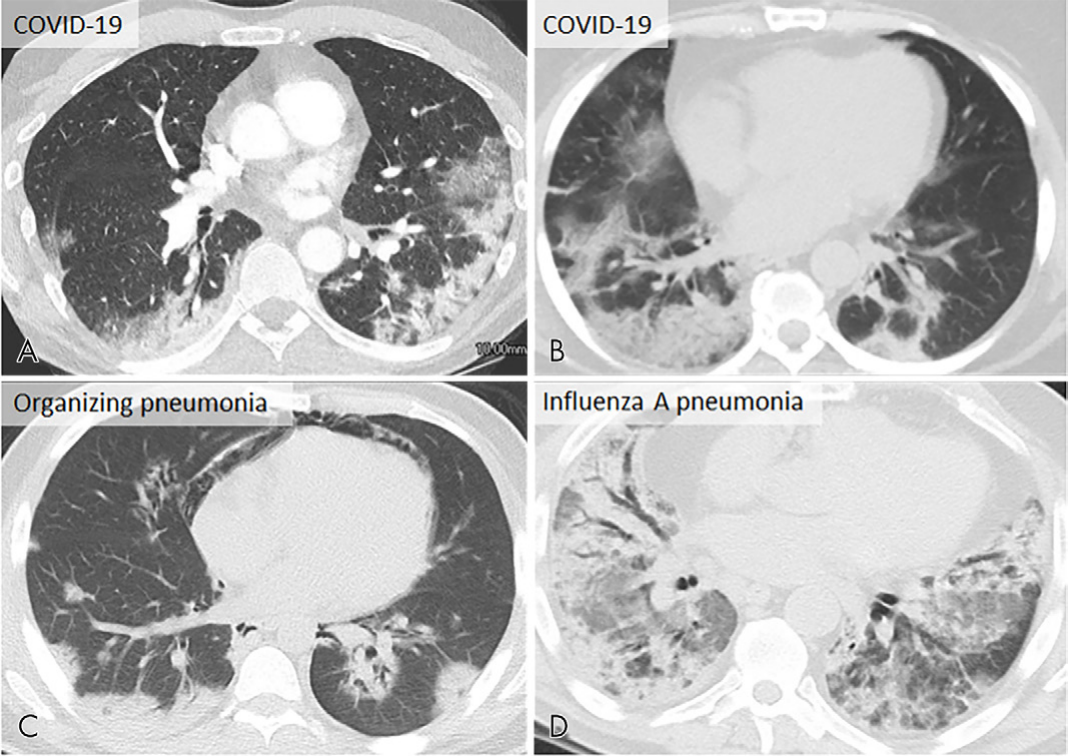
Typical CT imaging features for COVID-19 and other diseases with similar findings. Posterior, peripheral, and rounded GGO and consolidation in axial images of four patients; COVID-19 (*A, B*), organizing pneumonia secondary to dermatomyositis (*C*), and influenza A pneumonia (*D*). Organizing pneumonia and influenza pneumonia can be indistinguishable from COVID-19 by CT. Routine screening CT for diagnosis or exclusion of COVID-19 is currently not recommended by most professional organizations or the U.S. Centers for Disease Control and Prevention.
